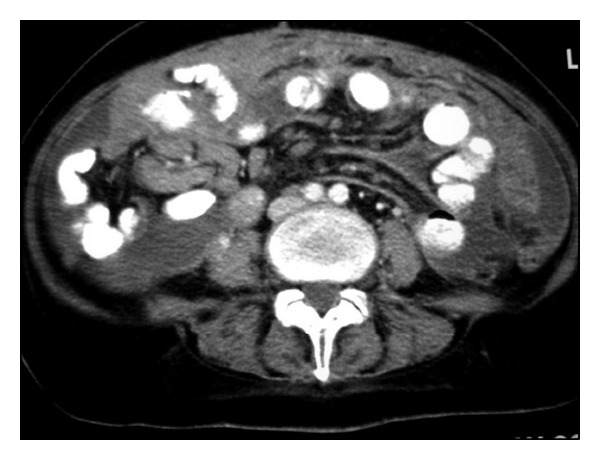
Patient with peritoneal lymphomatosis. Axial contrast-enhanced CT image shows ascites and infiltration of mesenteric fat.
